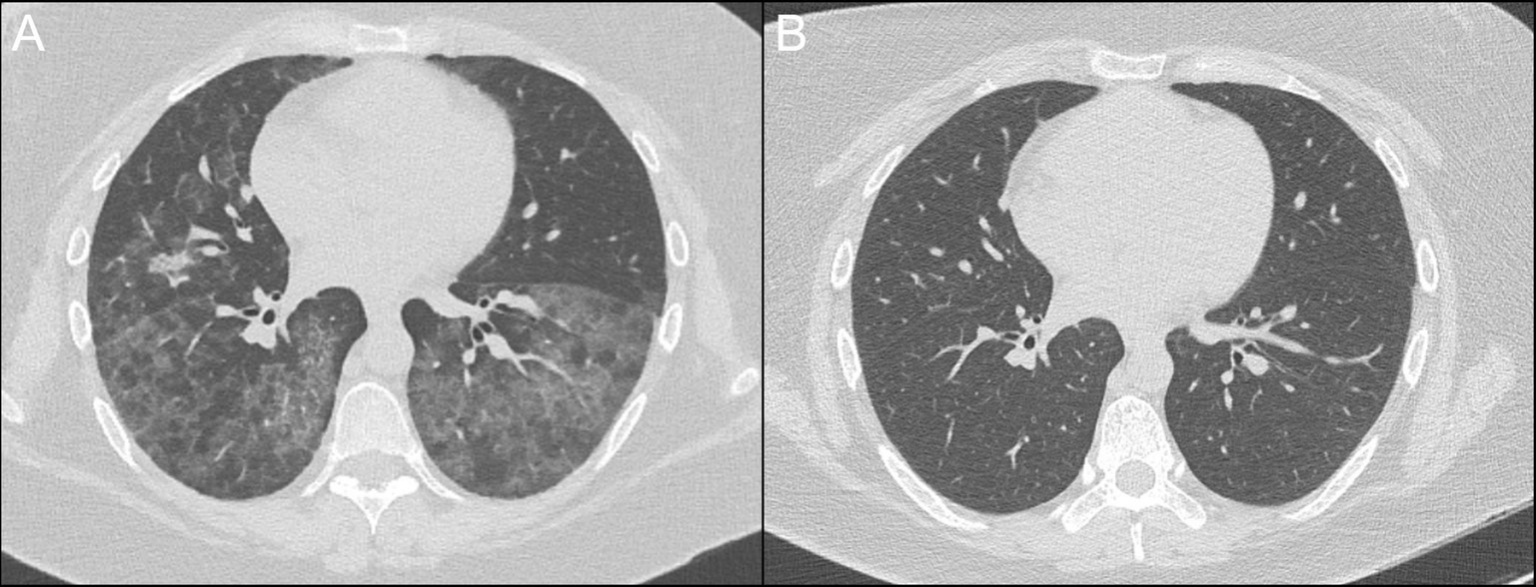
CT chest axial slices. (A) 8‐weeks post‐bilateral whole lung lavage demonstrating recurrence of bilateral ground glass opacities. (B) 5‐months post‐right lung lavage with sustained radiographic resolution of ground glass changes bilaterally.
